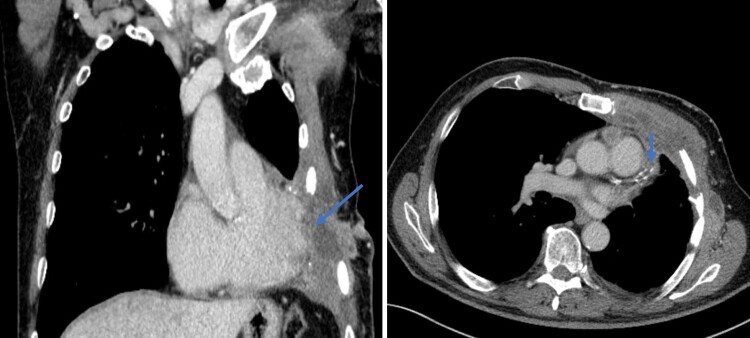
Displays a soft tissue window of a CT scan of the thorax, showing both a coronal and axial section. The images reveal a large malignant tumour infiltrating the myocardium of the left ventricle with contrast enhancement.
